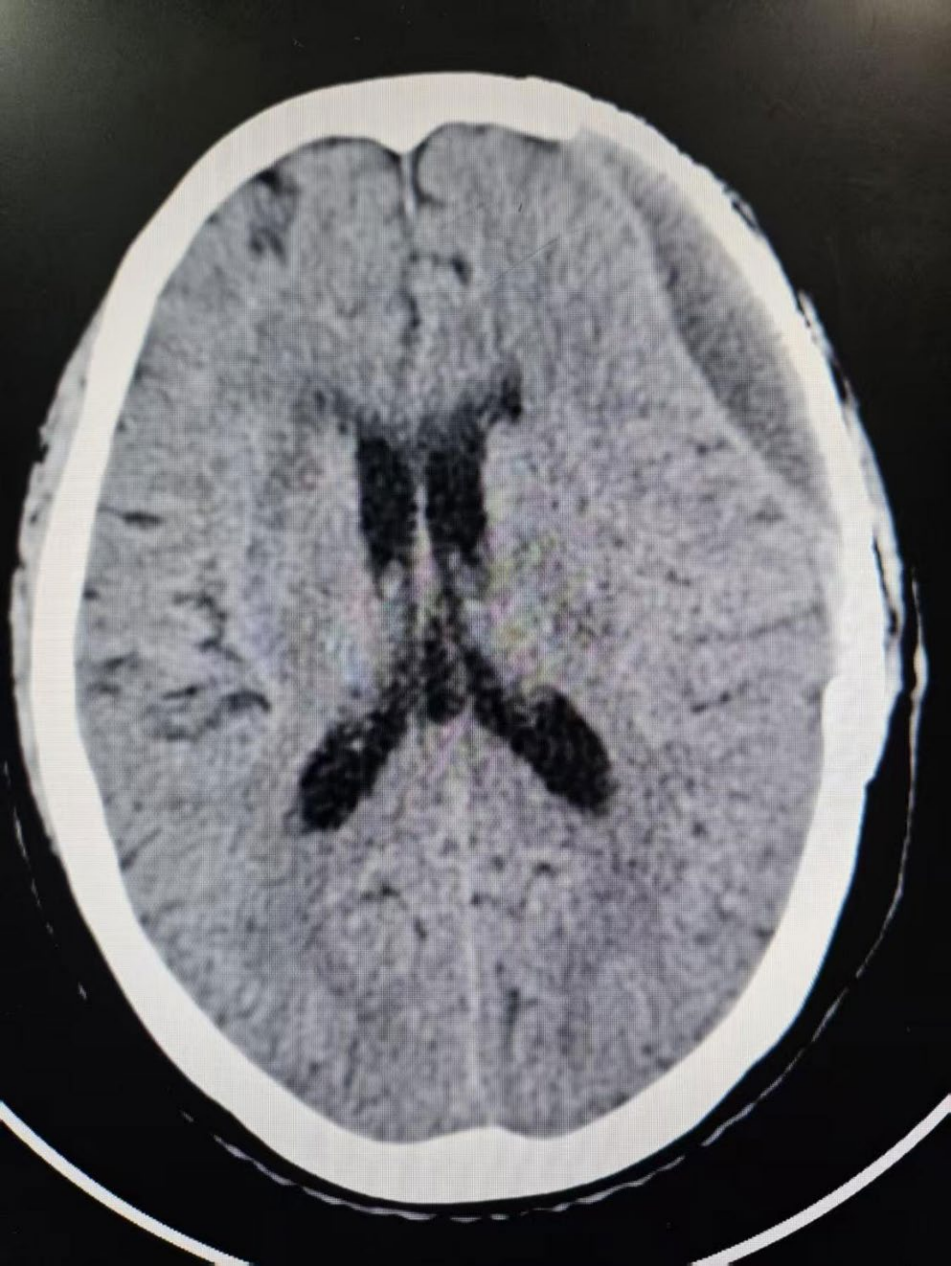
Epidural fluid collection (EFC) was identified based on low-density fluid in the epidural space on brain CT scans following CP
